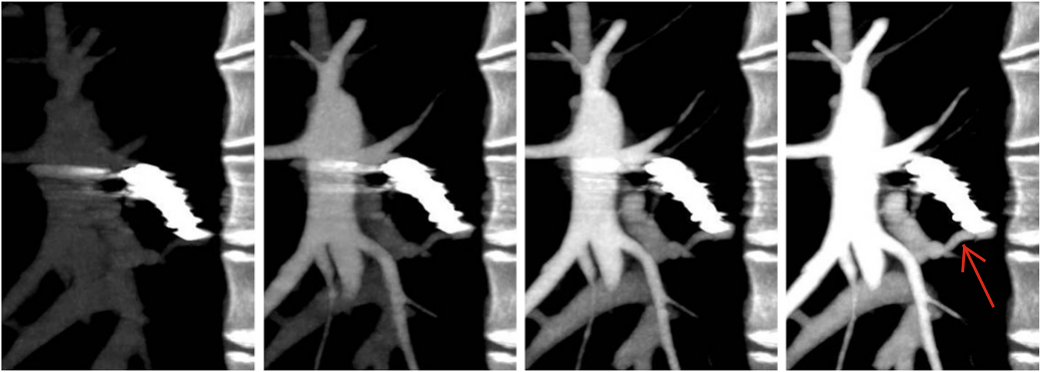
Dynamic CTA after the secondary intervention. No early contrast filling in the aneurysmal sac of PAVF was noted. The aneurysmal sac of PAVF was enhanced at the same phase of the enhancement of pulmonary vein (arrow).
